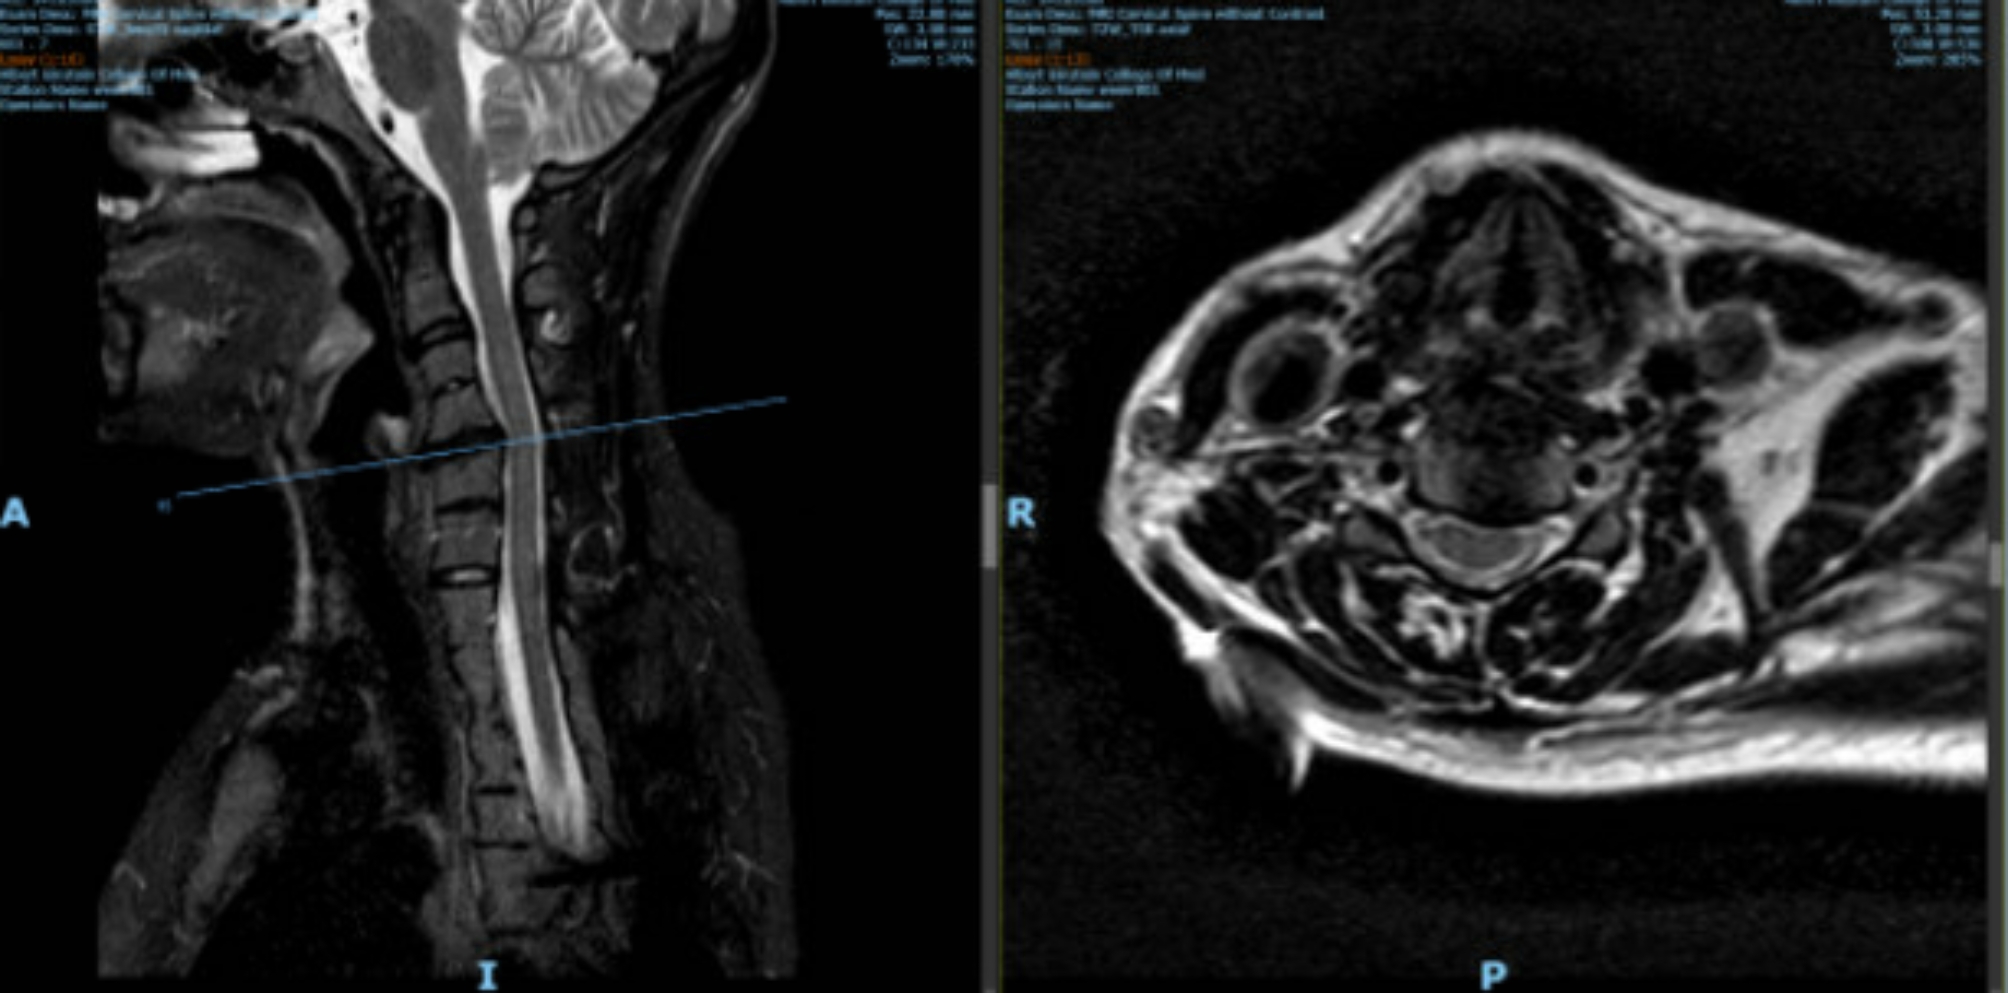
MRI of the cervical spine was significant for multilevel degenerative changes
